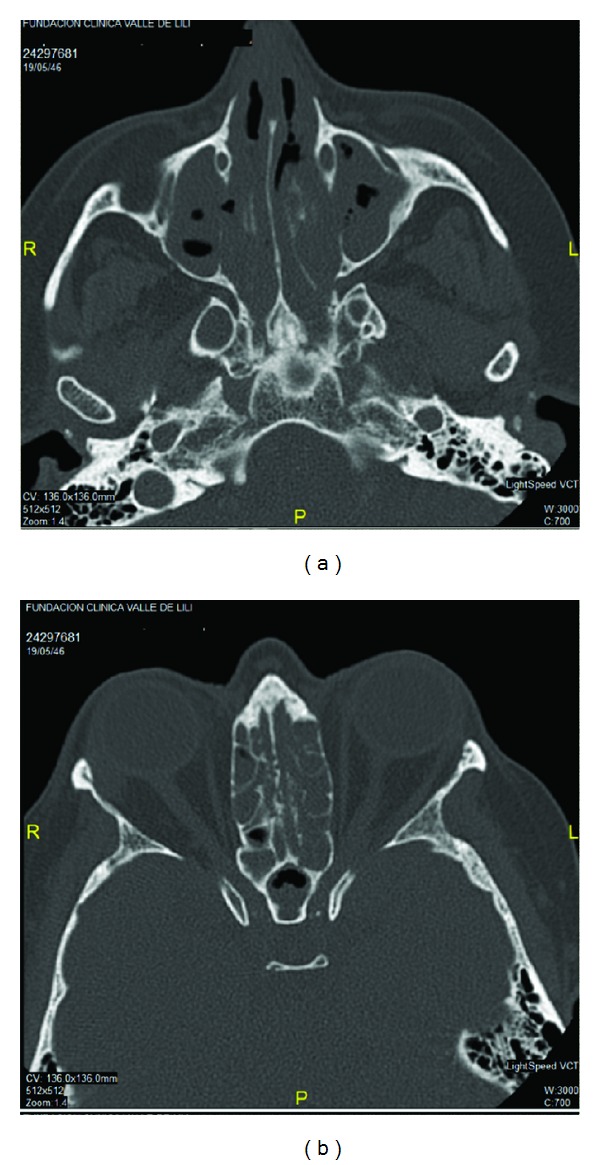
CT showing a diffuse sinus disease without erosions in a patient with granulomatosis with polyangiitis.
